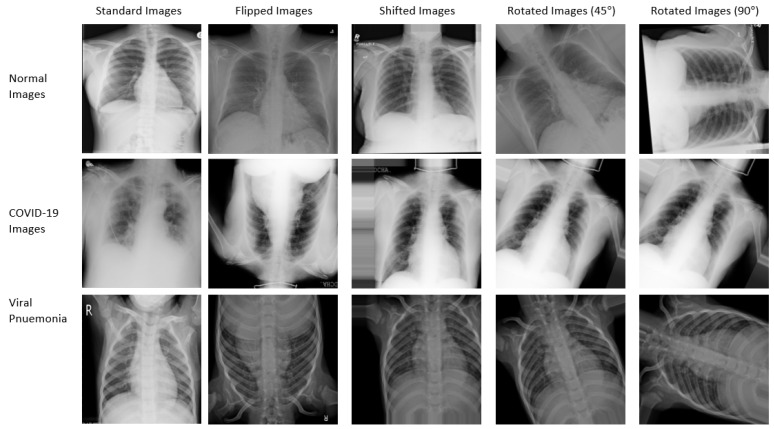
Samples of standard and augmented X-ray images used for training.
